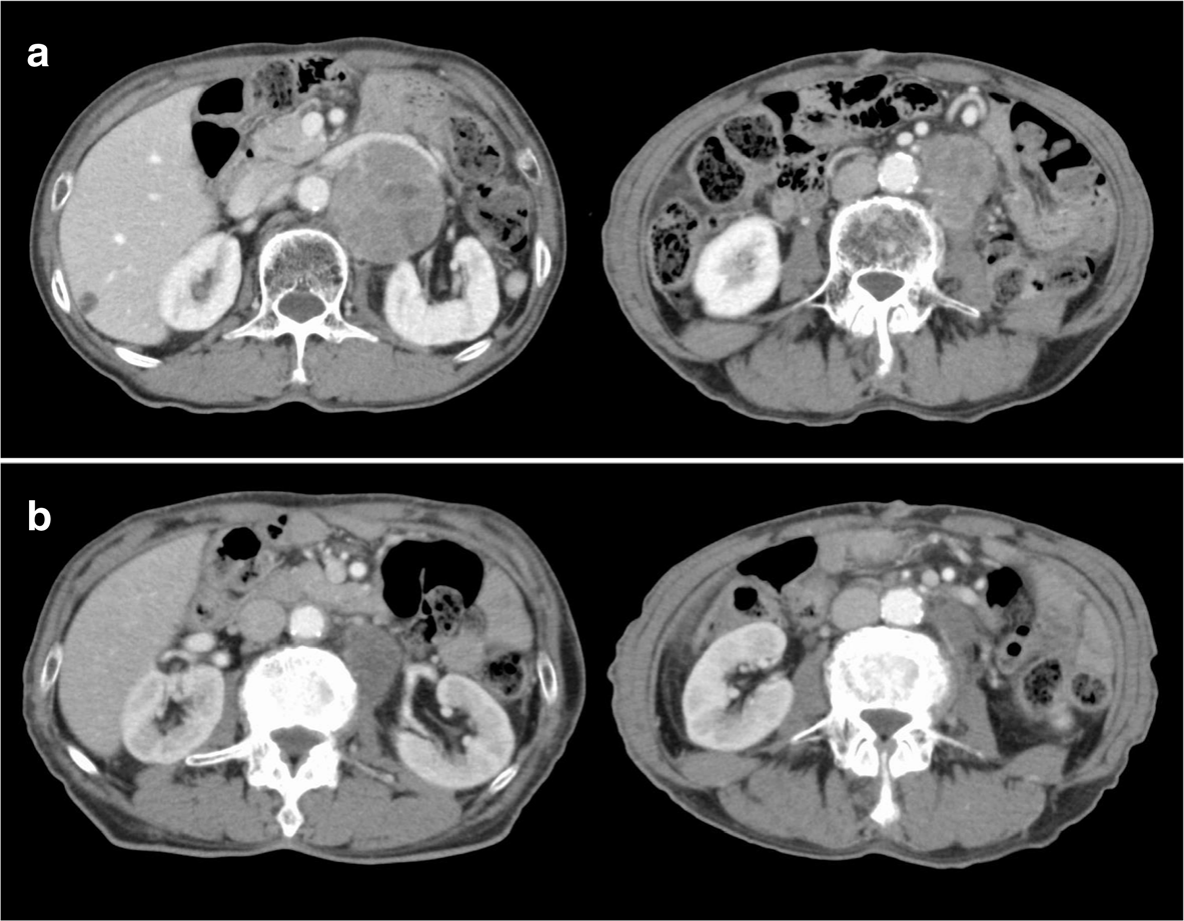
Computed tomography (CT) findings. a Abdominal contrast-enhanced CT before chemotherapy showed that the para-aortic lymph nodes were swollen (No. 16a2 70 mm, No. 16b1 30 mm respectively). CT after chemotherapy revealed that they were reduced in size (No. 16a2 (b))
